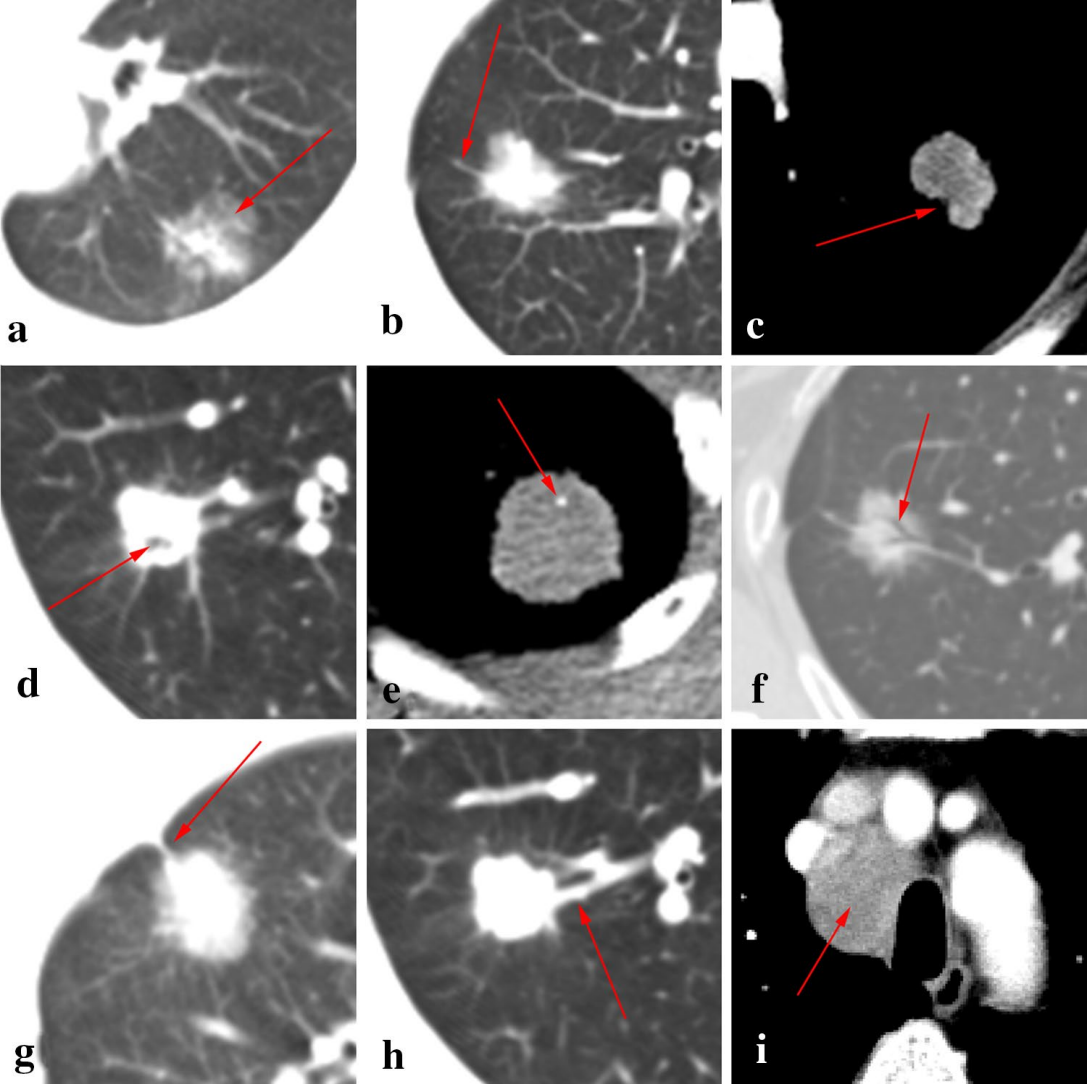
CT features. a The halo sign is a CT finding of ground-glass opacity surrounding a nodule or mass. b The spiculated sign is when the edge of a nodule or mass extends to the surrounding lung parenchyma, which contains linear strands that extend into the tissue of the lung but not into the pleural margin. c The lobulation sign is when the edge of the nodule shows uneven lobulated contour. d The cavity sign is a gas-filled space, seen as a lucency or low-attenuation area, within a pulmonary consolidation, mass, or nodule. e The calcification showed high attenuation, the average CT value of non-enhanced CT is >100 Hu. f The air bronchogram is a pattern of air-filled (low-attenuation) bronchi on a background of an opaque (high-attenuation) airless lung. g Pleural indentation is showed as a tapered or linear extension of the lesion to pleura, reflect the pulmonary fibrosis with adjacent pleural retraction. h The bronchovascular convergence sign is when one or more vessels reach the edge of the tumor or cross the tumor. i Lymphadenectasis means the size of a mediastinal or hilar lymph node is >1 cm in *short axis* diameter
